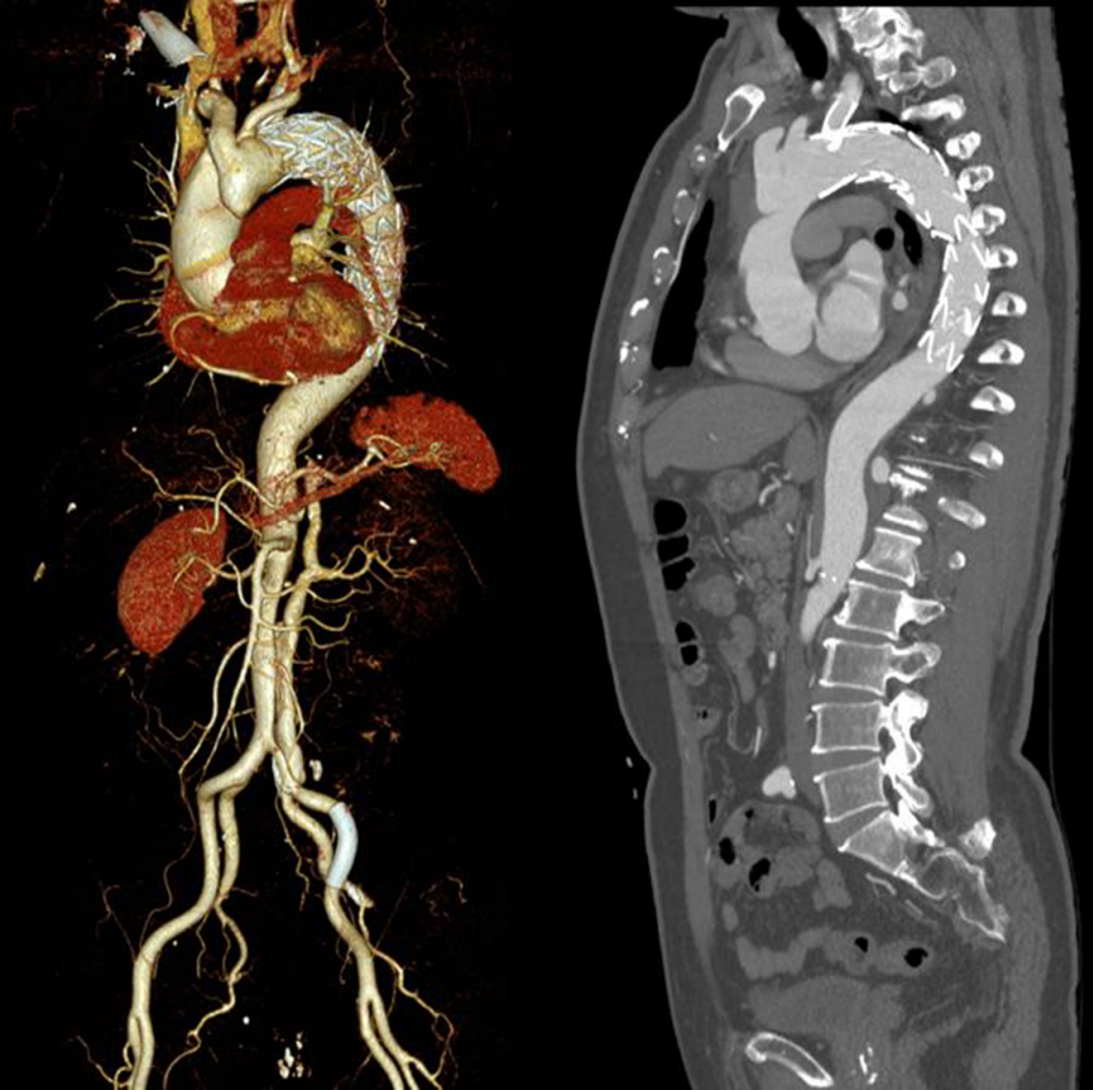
Follow up examination of aortic CTA.
